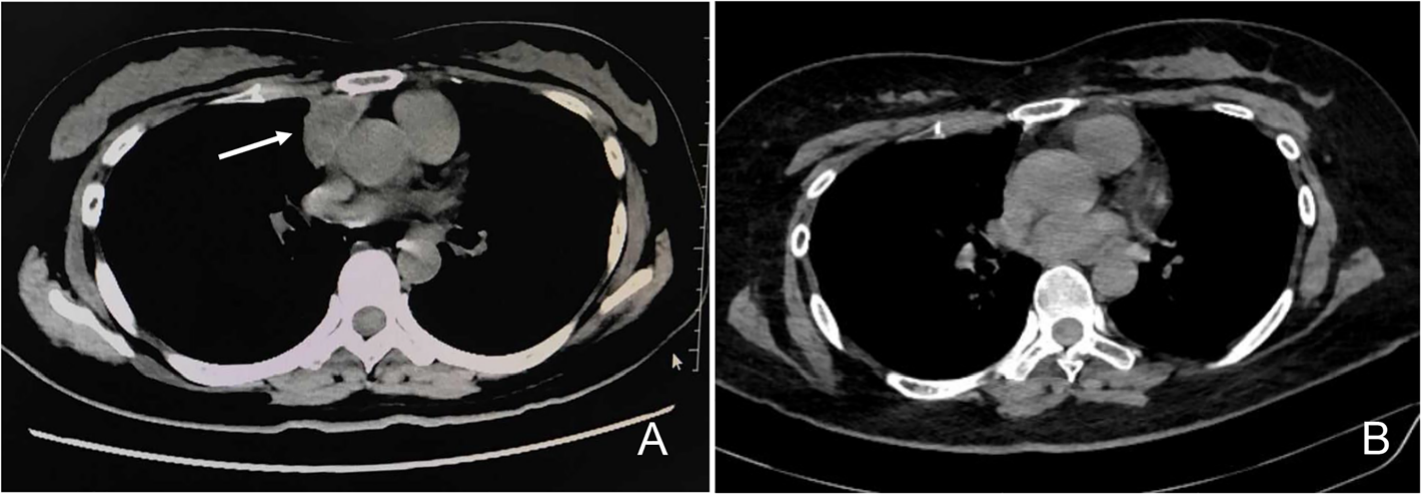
Chest CT showed a 3.1 cm × 1.9 cm thymoma on August 3, 2016 (a) and was normal without thymoma recurrence after thymectomy on July 11, 2018 (b)
